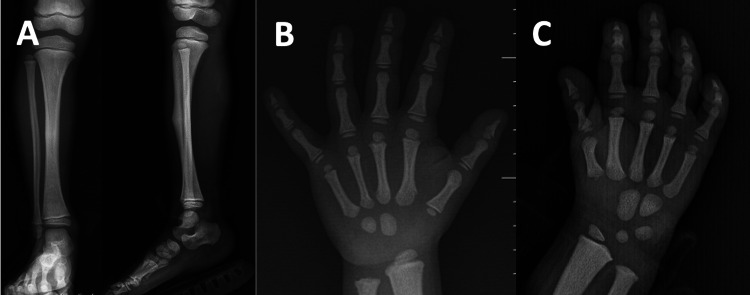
Radiological findings of the first, second, and third cases are being represented. A. Radiological findings of the first case. Osteosclerosis in the tibia and fibula, as well as healing fracture on the tibia can be noted. B. Radiological findings of the second case. Radiograph of the left hand shows osteosclerosis and acro-osteolysis of the distal phalanx. C. Radiological findings of the third case. Radiograph of the left hand shows osteosclerosis and acro-osteolysis of the distal phalanx.
